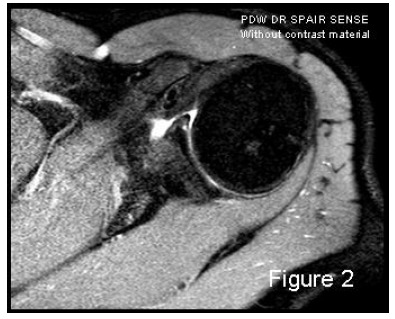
An example of MRI image that takes advantage of joint effusion as contrast material in acute scenario.
